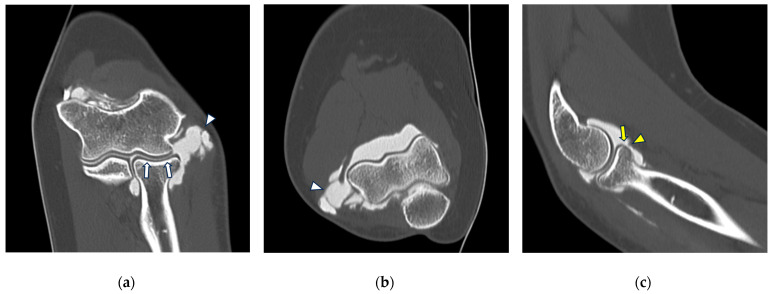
CT arthrography of a patient with lateral epicondylitis subjected to multiple corticosteroid injections. (a,b) Coronal and axial images show extravasation of intra-articular contrast into lateral periarticular soft tissues through a large full-thickness tear of both the radial collateral ligament and the proximal common extensor tendon (white arrowheads). Diffuse thinning of radial head dish cartilage is also displayed (white arrows); (c) sagittal image also shows distal displacement of the annular ligament (yellow arrowhead) and cartilage fraying of the radial head side (yellow arrow).
